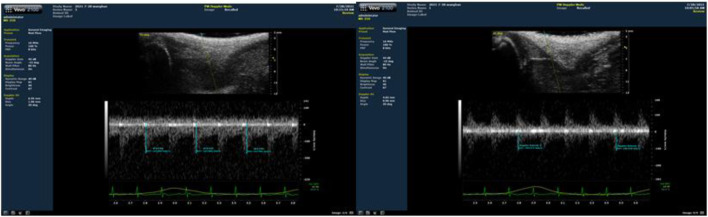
Ultrasound detection of the proportion of blood flow restriction (The left picture is before blood flow restriction, and the right picture is after restriction).
